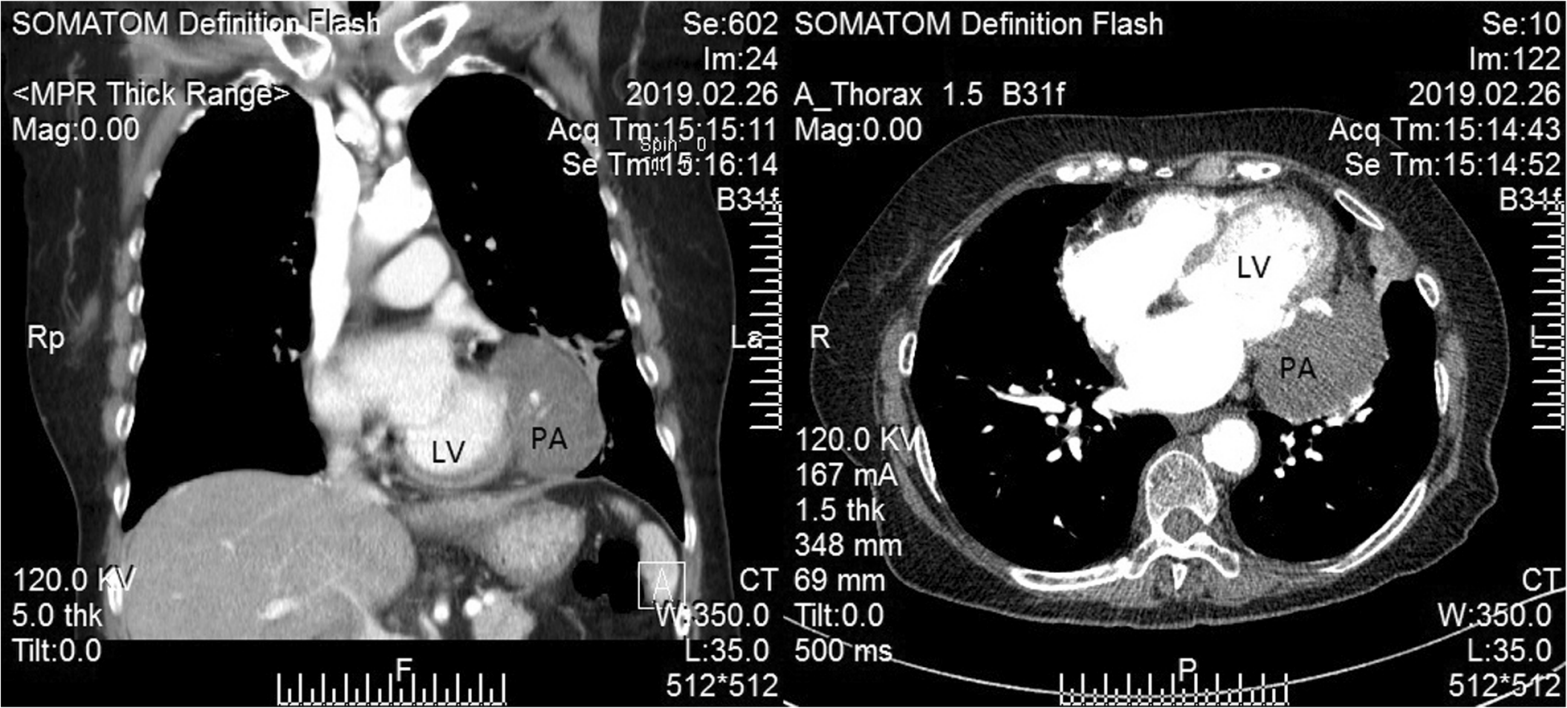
The result of Cardiac multislice computed tomography (MSCT) confiemed the formation of the pseudoaneurysm and thrombus filling with pseudoaneurysm. (PA: pseudoaneurysm)
